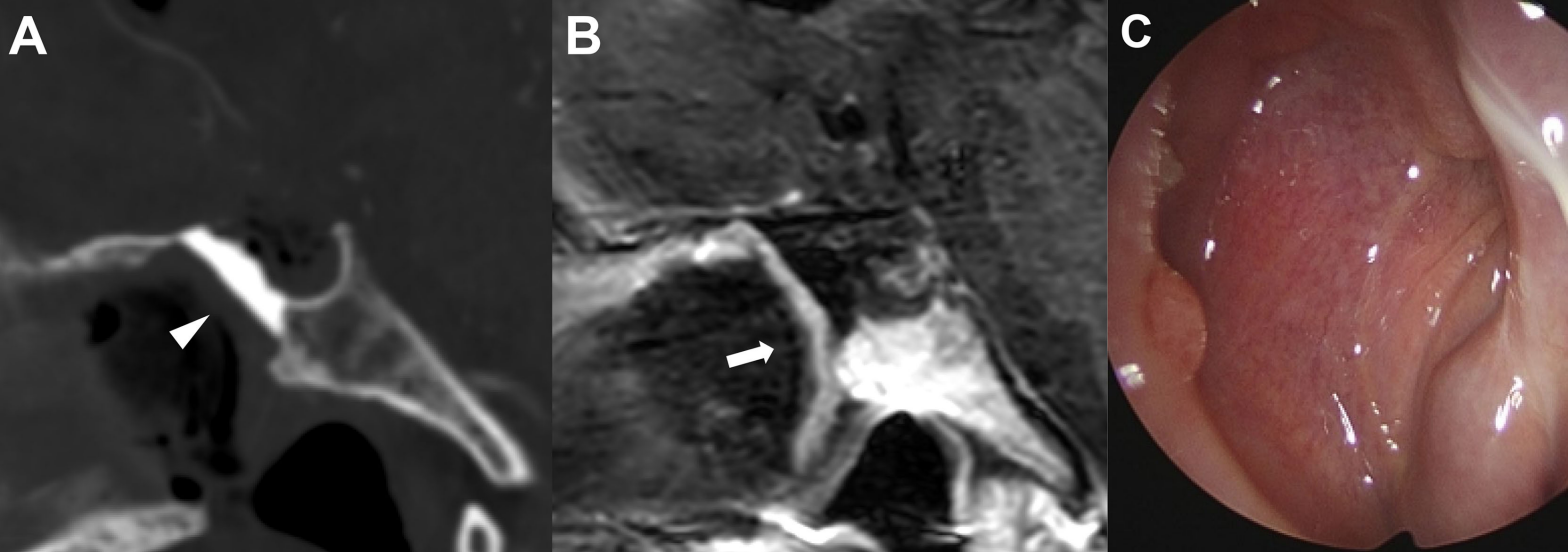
Representative images of skull base reconstruction in endoscopic endonasal surgery of craniopharyngioma. (A) Postoperative sagittal computed tomography image of the operative area with hydroxyapatite *in situ* (arrowhead). (B) Postoperative sagittal magnetic resonance imaging with contrast enhancement indicating the integrity of vascularized naso-septal flap (arrow). (C) Complete sealing status of hydroxyapatite by the flap 3 months after surgery.
